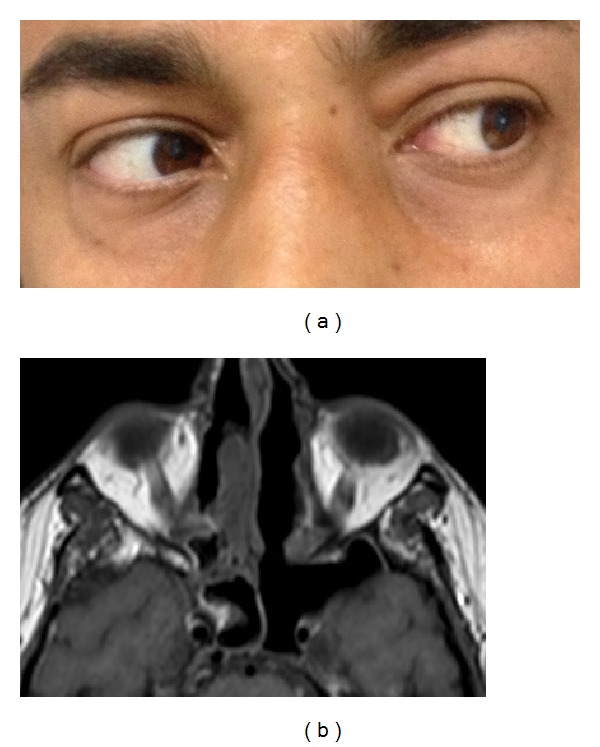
(a) Photo of the patient at three months after ESS showing complete recovery from left abducens nerve palsy. (b) Axial MRI performed one month after surgery. Enlargement of the left sphenoid sinus and resolution of the disease can be seen.
